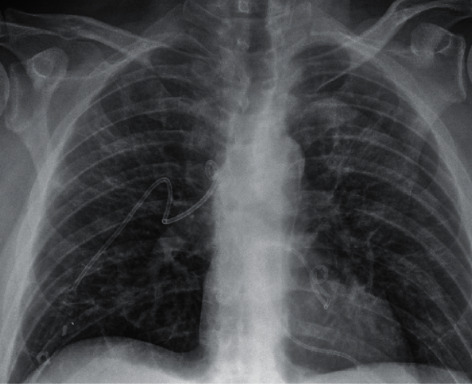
Anteroposterior chest radiograph confirming chest tube placement.
